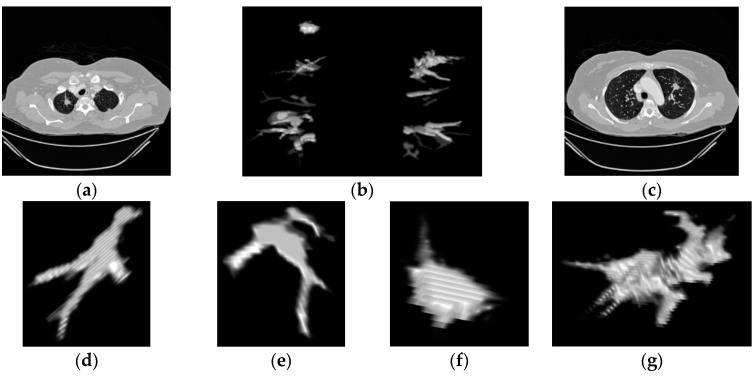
Precise Lung nodules detection (a) CT image containing the first nodule; (b) Lung 3D reconstruction; (c) CT image containing the second nodule; (d–g) all possible candidates before classification; (d,e) Non-nodules; (f,g) lung nodules determine after classification.
